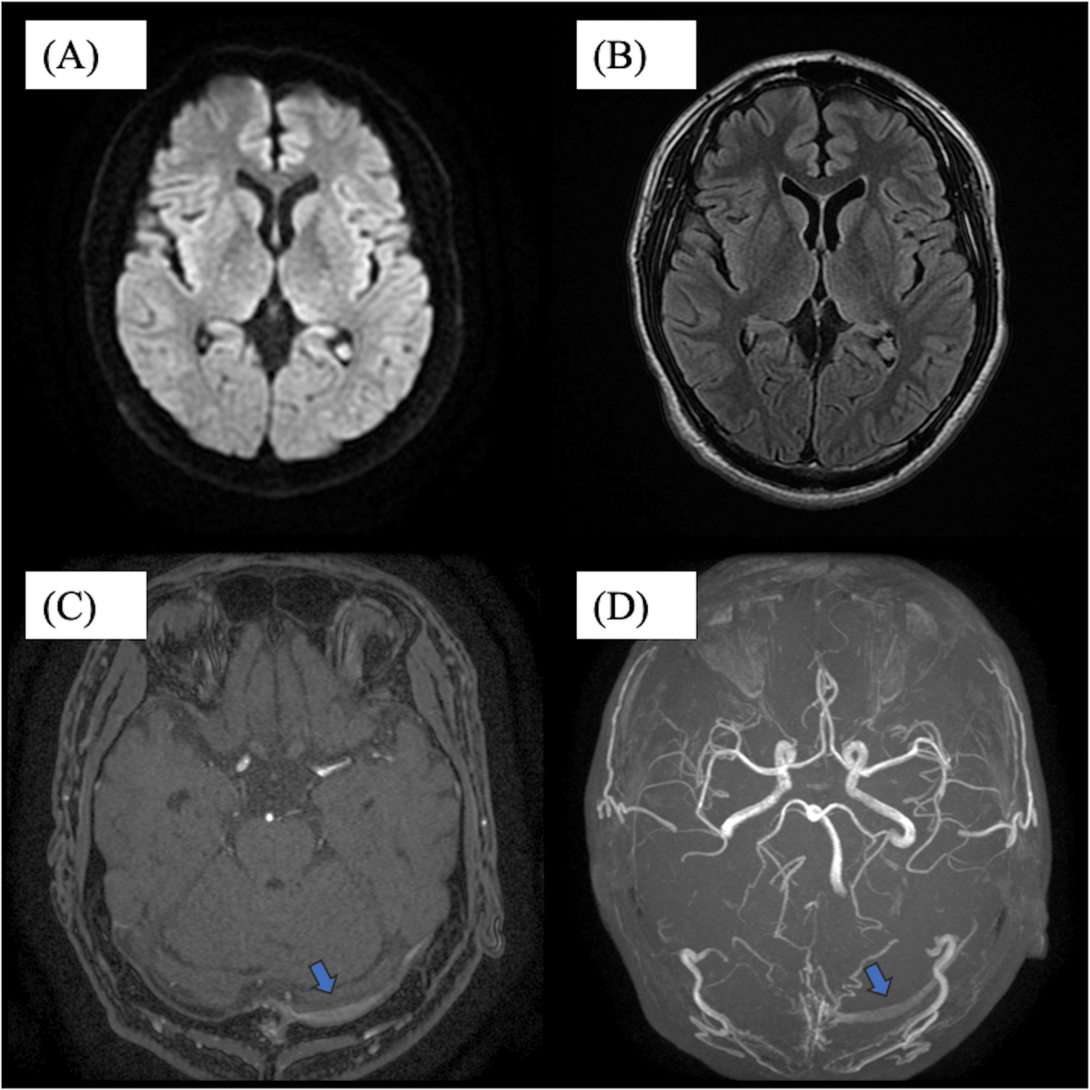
MRI and magnetic resonance angiography (MRA) showing abnormal vascular signals in the left transverse sinus. (A) MRI diffusion-weighted imaging (DWI) showed no abnormal findings. (B) MRI fluid-attenuated inversion recovery (FLAIR) showed no abnormal findings. (C) Time-of-flight (TOF) MRA axial scan showing abnormal flow-related signal in the left transverse sinus (arrows). (D) TOF MRA 3D reconstruction showing abnormal flow-related signal in the left transverse sinus (arrows).
